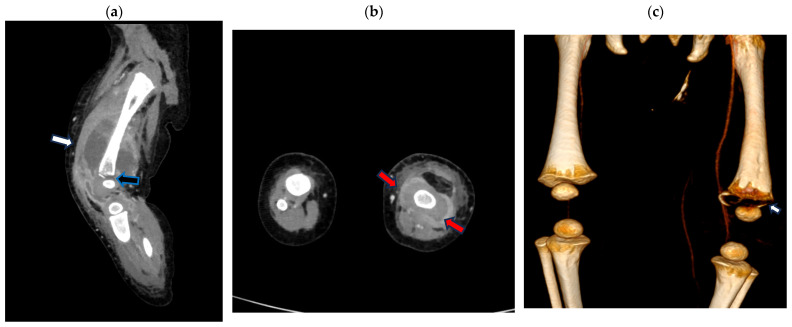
Contrast-enhanced computed tomography (CT) scan of the left leg (a) sagittal multi-planar (MPR) reconstruction: left fluid collection surrounding the distal metaphyseal femoral bone (white arrow) extending to the joint with the cortical erosion/destruction of the metaphyseal distal bone (black arrow); (b) axial MPR reconstruction: left fluid collection surrounding the distal metaphyseal femur (red arrow); (c) volume rendering CT reconstruction bone window demonstrating distal metaphyseal bone erosion (arrow).
